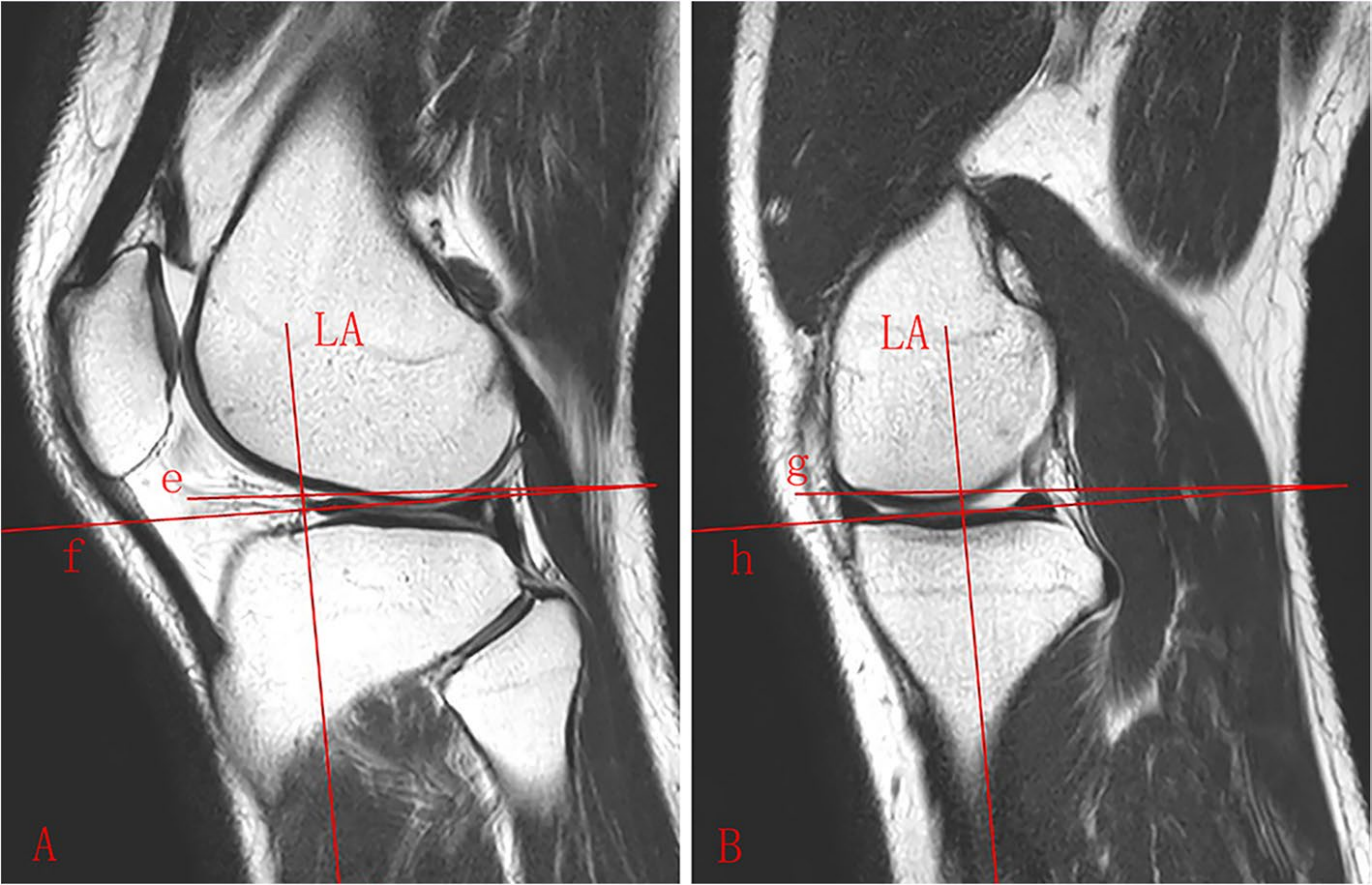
Lateral and medial MS. A sagittal MRI image shows the anterior and posterior horns of lateral meniscus clearly. The LA is the longitudinal axis of the proximal tibia, line f is perpendicular to LA, and line e joints the highest points of the anterior and posterior horns of lateral meniscus. The lateral MS is calculated as the angle between line e and line f. B sagittal MRI image shows the anterior and posterior horns of medial meniscus. Line h is perpendicular to LA, and line g joints the highest points of the anterior and posterior horns of medial meniscus. The medial MS is calculated as the angle between line g and line h
